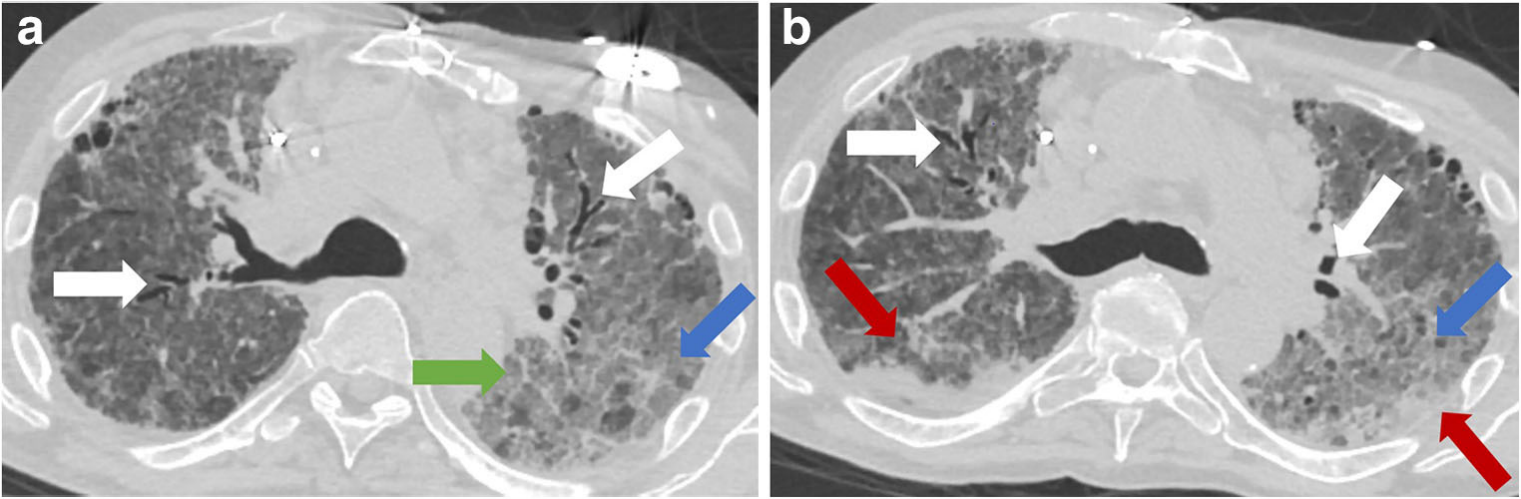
Progressive stage COVID-19 (5–8 days): Axial CT of the chest demonstrates diffuse distributionof GGO (blue arrows in A and B), crazypaving pattern (green arrow in A), consolidations (red arrows in B), and bronchiectasis (white arrows).
